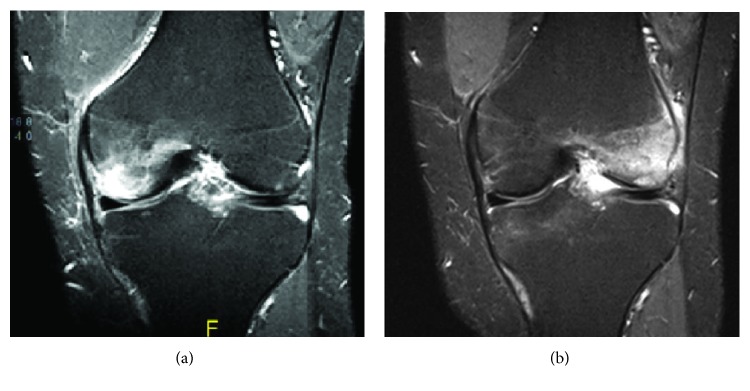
MRI of the left knee (a) in September 2016 and (b) three months later.
